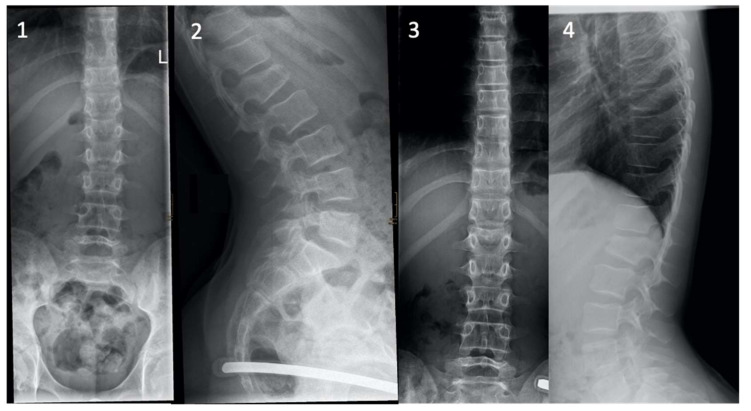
Images 1 and 2 show the radiographs of an A1.2 L1 fracture in a 12-year-old girl sustained after falling off a trampoline. The patient was treated conservatively by receiving adequate analgesia and sports abstinence. The radiographs at the last follow-up after three months of therapy show no further dynamics and a healed fracture (Images 3 and 4).
